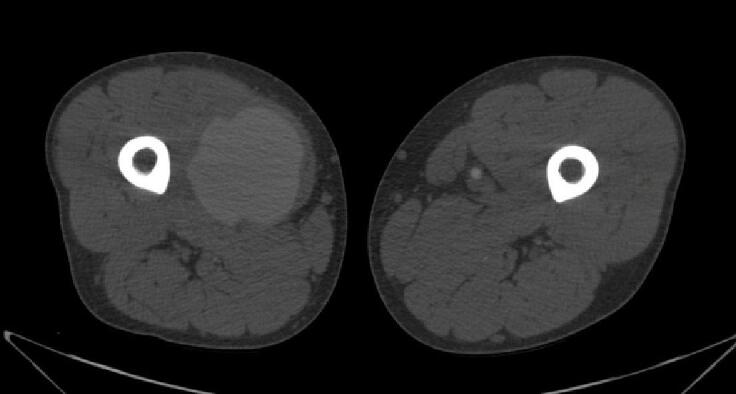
A Computed Tomographic Angiography (CTA) of the Lower Extremities: Axial view demonstrating a giant pseudoaneurysm of the right superficial femoral artery (SFA).
